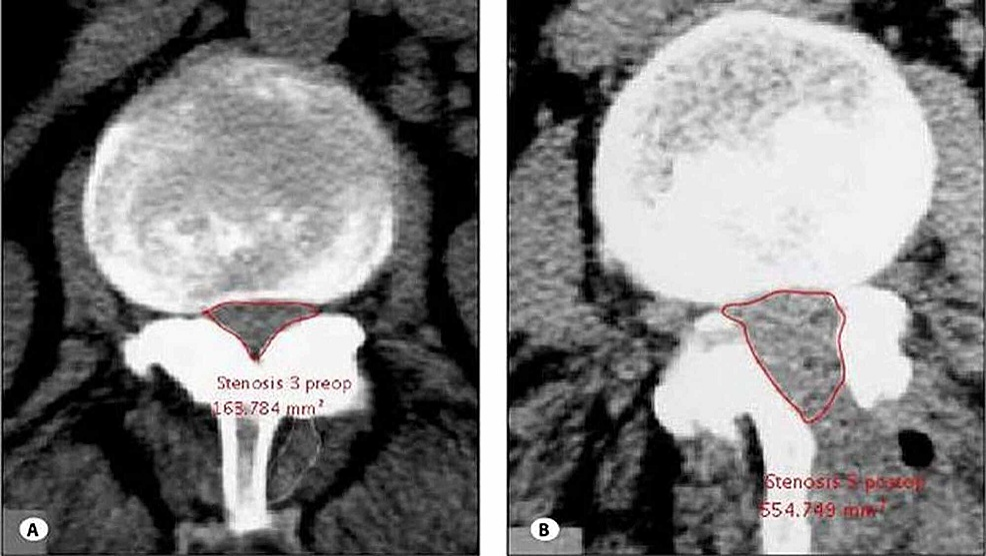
Preoperative (a) and postoperative (b) radiographs showing decompression of the central canal after a unilateral laminectomy and bilateral decompression. Figure reproduced with permission from Yaman et al. [16].
